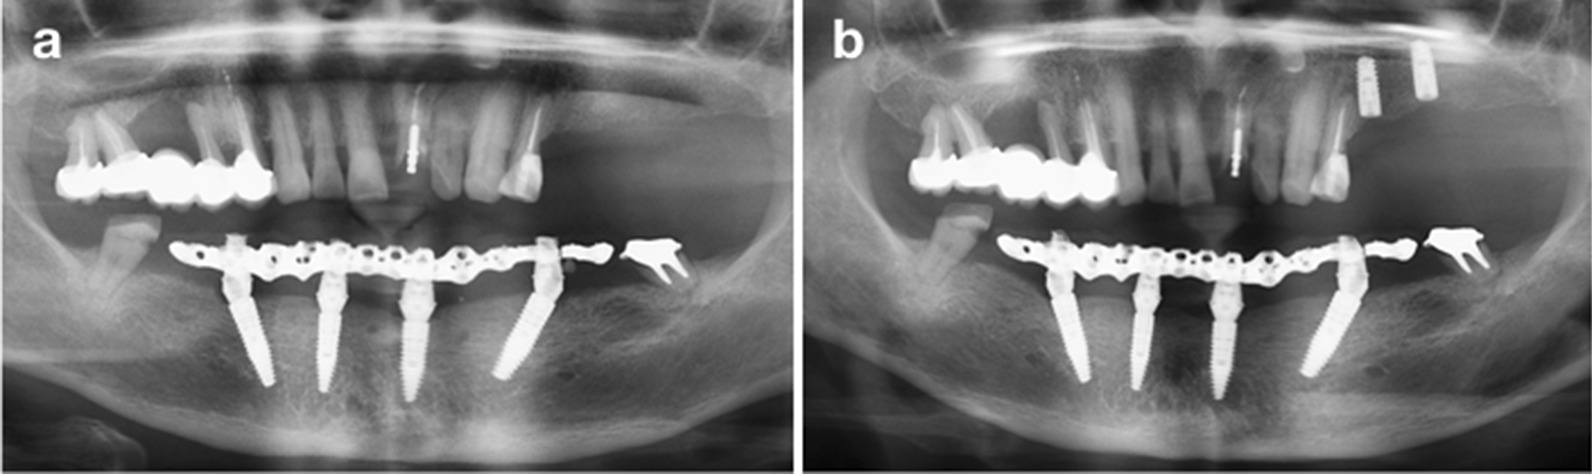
Radiographs. a Panoramic radiograph after implant surgery and immediate loading. b Panoramic radiograph at the 6-month follow-up (before definitive restoration): stable marginal bone levels were shown
